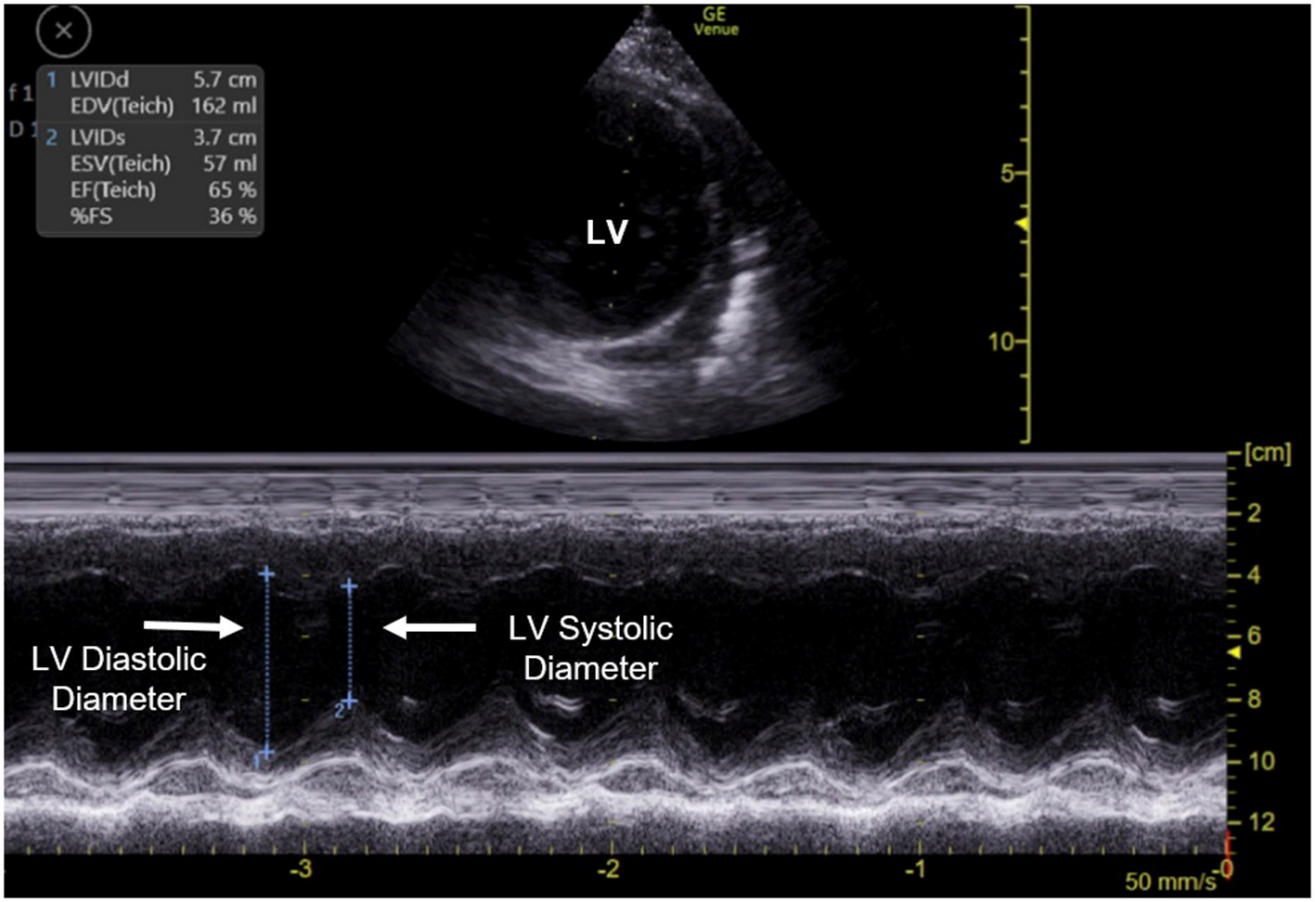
Quantitative estimation of left ventricle (LV) function using fractional shortening. The upper half of the image displays the placement of M-mode line through the left ventricle in a parasternal short axis view of the heart. The lower half of the image displays the M-mode output. Left ventricle systolic and diastolic diameters are measured to calculate fractional shortening. The scale represents the depth of imaging. LV, Left ventricle.
